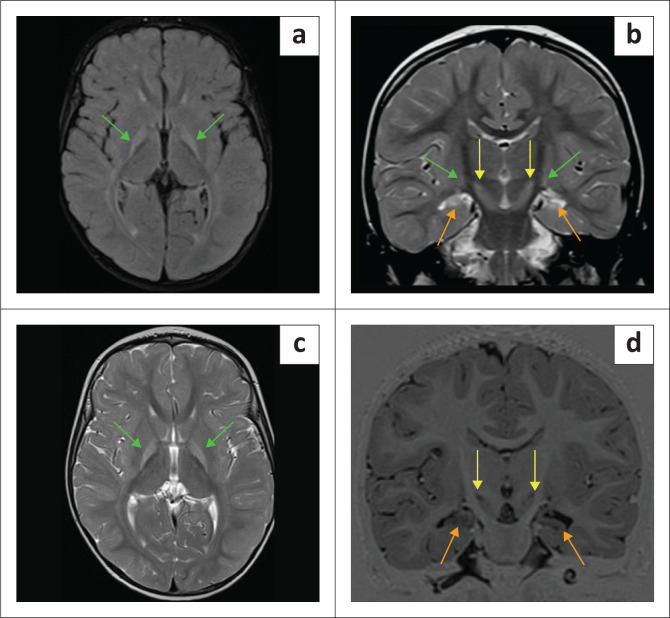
(a-d) Follow-up MRI in a 22-month-old male child with dyskinetic cerebral palsy and sensorineural hearing loss. There is severe bilateral hippocampal atrophy from intractable seizures. The hyperintense signal at the globi pallidi (green arrows), hippocampi (orange arrows) and both subthalamic nuclei (yellow arrows) are unchanged from the neonatal study and indicative of *kernicterus*. Note relative sparing of thalami and posterior putamina, which are expected to be involved in the Rolandic basal ganglia-thalamus subtype of hypoxic-ischaemic brain injury.
